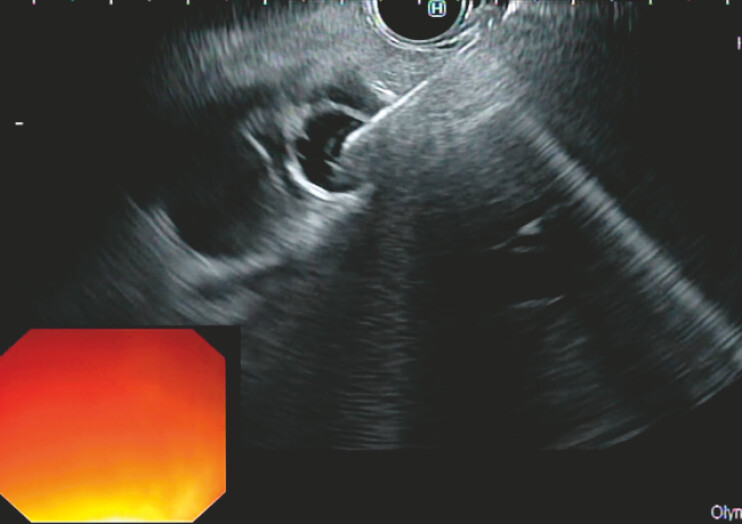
Puncture of the dilated bile duct at the cystic confluence under endoscopic ultrasound guidance.
